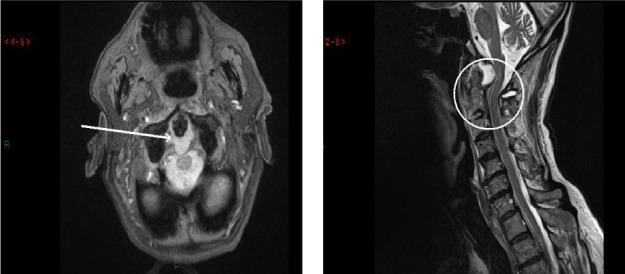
Magnetic resonance imaging: The spinal cord appears to be compressed by a collection of fluid located behind the odontoid peg.
